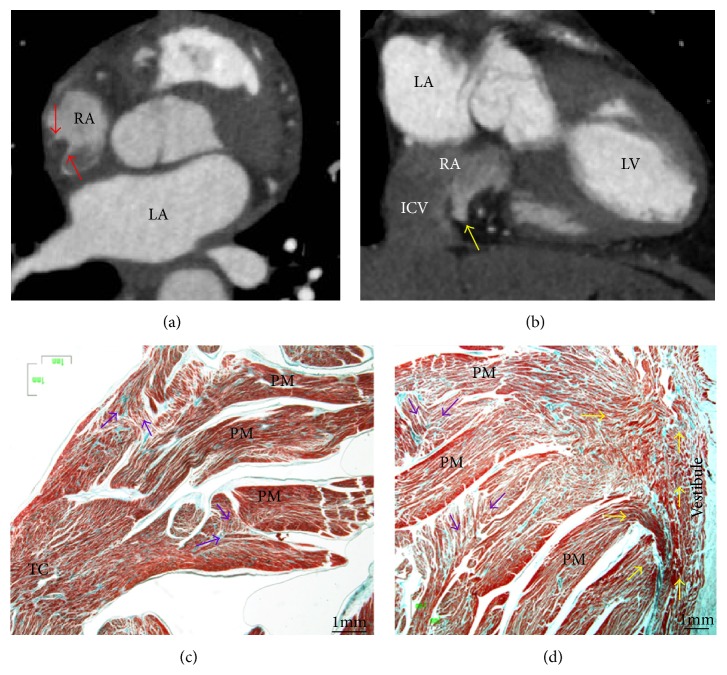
(a) Axial CT angiogram shows a prominent crista terminalis (red arrows) in the right atrium. (b) Coronal CT shows a sub-Eustachian pouch (yellow arrow) in the median aspect of the right inferior cavoatrial junction. (c, d) Frontal sections through the terminal crest at the origin and ending of the pectinate muscles. Note the irregular alignment (purple arrows) of the muscular myofibrils within the pectinate muscles and between them and the circumferentially arranged myocytes in the vestibule (yellow arrows). ICV = inferior cava vein, LA = left atrium, LV = left ventricle, PM = pectinate muscles, RA = right atrium, and TC = terminal crest.
